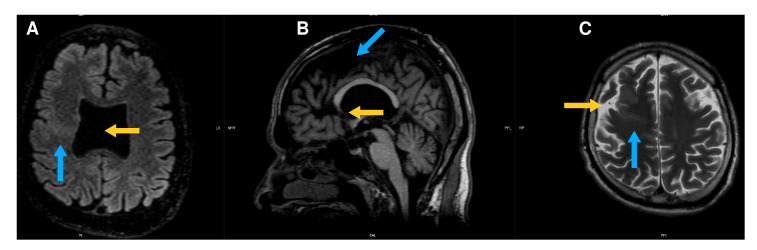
Hallazgos en la resonancia magnética de recuperación de la inversión atenuada de fluido (FLAIR). A) Corte axial potenciado: se observa sustancia gris anormal en el lóbulo frontal derecho, la cual se extiende desde la periferia hasta la pared del ventricular. No se visualiza el *septum pellucidum.*
